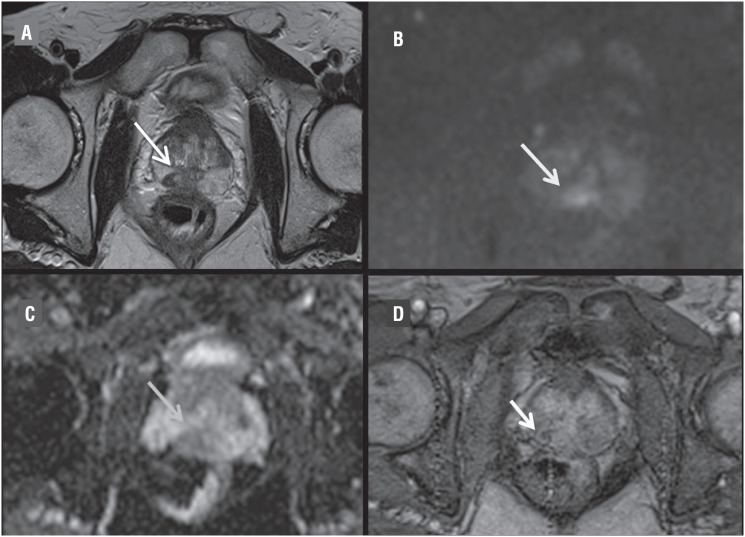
A) Axial T2-weighted image with a round hypointense focal lesion (6mm) in the right posteromedial peripheral zone at the midgland level (arrow). B) On diffusion-weighted imaging with high b value (1000), a focal markedly hyperintense lesion (arrow), with markedly hypointense (arrow) value on ADC map (0.8×10-3mm2/s), consistent with a PI-RADS 4 lesion. c) is described. On Dynamic contrast Enhanced image an enhancement (arrow) of nodular lesion is showed (D).
